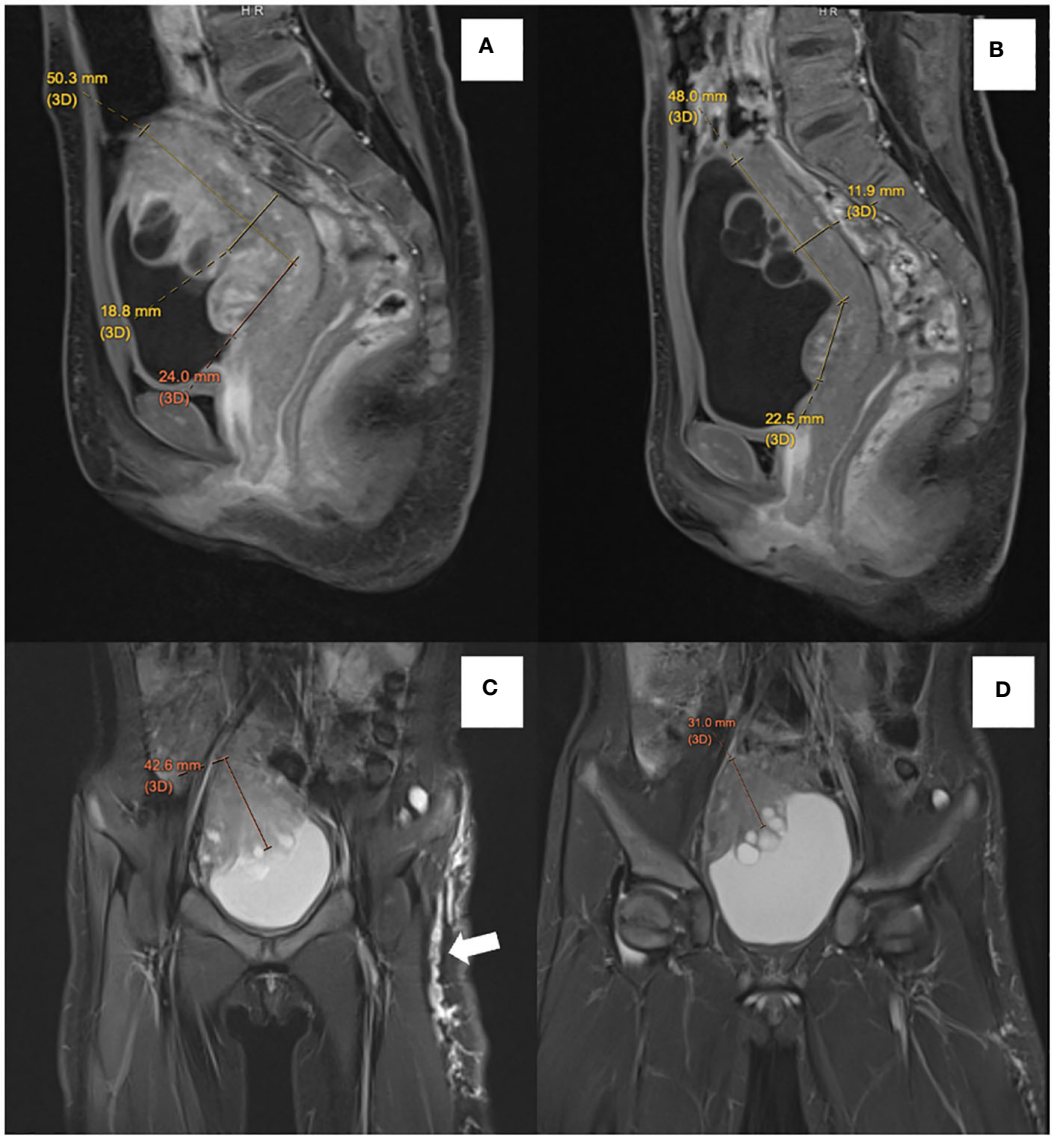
Comparison of sagittal postcontrast T1-weighted images before treatment (A) with follow-up MRI (B) showed reduced thickness of the solid component of ganglioneuroma from 18.8 mm to 11.9 mm. There is also an interval reduction in the degree of enhancement of solid components. Contrasting coronal T2-weighted short-tau inversion recovery (STIR) before treatment (C) with follow-up MRI (D) showed a reduction in thickness of the solid component of ganglioneuroma from 42.6 mm to 31.0 mm. Multiple subcutaneous neurofibromata along the left lateral thigh (white arrow) also showed an interval reduction in size and extent in the follow-up MRI.
